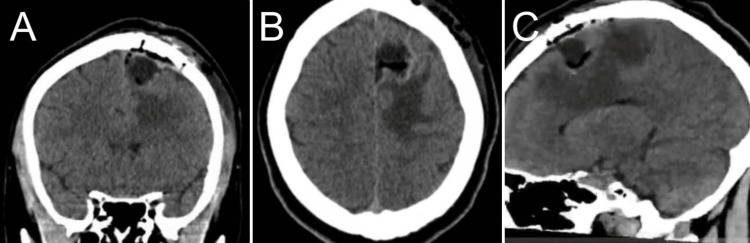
Postoperative CT after tumor resection (A) Coronal, (B) axial, and (C) sagittal postoperative CT images demonstrating the surgical cavity after tumor resection. Confirming complete tumor resection without evidence of structural anatomical damage and a minimal frontal craniotomy with very minimal brain exposure. CT: computed tomography
